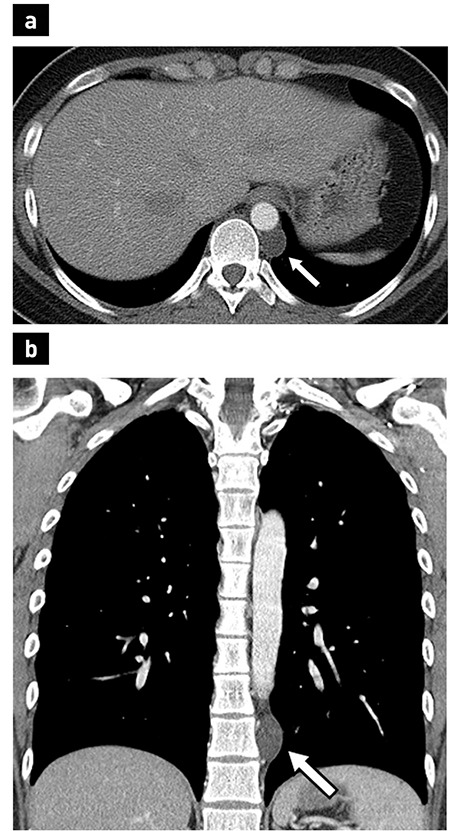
Contrast-enhanced axial (a), and coronal (b) chest computed tomography show a well-defined ovoid thin-walled cystic mass without enhancement (arrows) in the left paravertebral space adjacent to the tenth vertebra.
